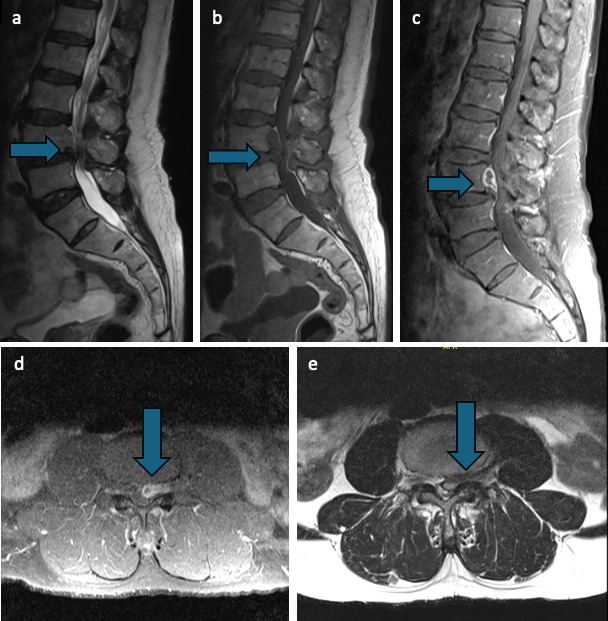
Preoperative magnetic resonance images of sagittal T2 (a), sagittal T1 (b), and sagittal T1 with contrast sequences (c) showing canal stenosis at the level L3-4 and L4-5 vertebrae, with migrating L4-5 disc material behind the L4 vertebral body compressing the left lateral recess and with peripheral contrast enhancement, and axial T1 with contrast (d) and axial T2 sequences (e) showing significant canal stenosis with left L4 foraminal stenosis.
